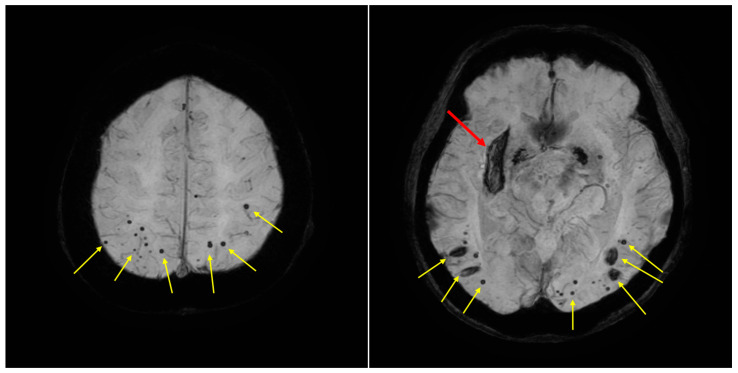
Brain Magnetic Resonance Imaging (MRI) Results. Susceptibility-weighted imaging (SWI) reveals multiple hypointense areas, including deep structures such as the right putamen (red arrow) and also distributed throughout lobar regions of the brain (yellow arrows). Given the presence of these multiple hemorrhages, both hypertensive small-vessel disease (HTN-SVD) and cerebral amyloid angiopathy (CAA) were considered in the differential diagnosis. However, the patient had no history of hypertension, and indeed, no instances of elevated blood pressure were noted during his hospitalization. This clinical information argued against a primary diagnosis of HTN-SVD. Attribution of these findings to either HTN-SVD or CAA should be made following standardized diagnostic criteria and careful evaluation of the lesion distribution patterns, which can distinguish between predominantly deep versus mixed lobar and deep microhemorrhages. Additionally, neuropsychological testing diagnosed him with dementia (Clinical Dementia Rating: 1). His cognitive decline and higher brain dysfunction may have contributed to his refusal to seek hospital care, resulting in the worsening of his gouty tophi. Hyperuricemia was associated with a significantly increased the risk of both stroke incidence (pooled RR, 1.42; 95% CI, 1.31–1.53) and stroke mortality (pooled RR, 1.53; 95% CI, 1.18–1.99) in meta-analyses [7]. For the risk of stroke mortality, the association between HUA and ischemic stroke (pooled RR, 1.39; 95% CI, 1.31–1.47) was more significant than that of hemorrhagic stroke (pooled RR, 1.13; 95% CI, 1.02–1.26) [4]. Additionally, hyperuricemia might be related to the progression of cerebral small vessel disease [8]. Consequently, in select cases with this severe phenotype—especially when accompanied by poor self-management—a stepwise approach beginning with cognitive screening and followed by neurologic evaluation as needed may help uncover the true barrier to effective treatment. These observations require confirmation in additional cases or a case series before broader recommendations can be made.
